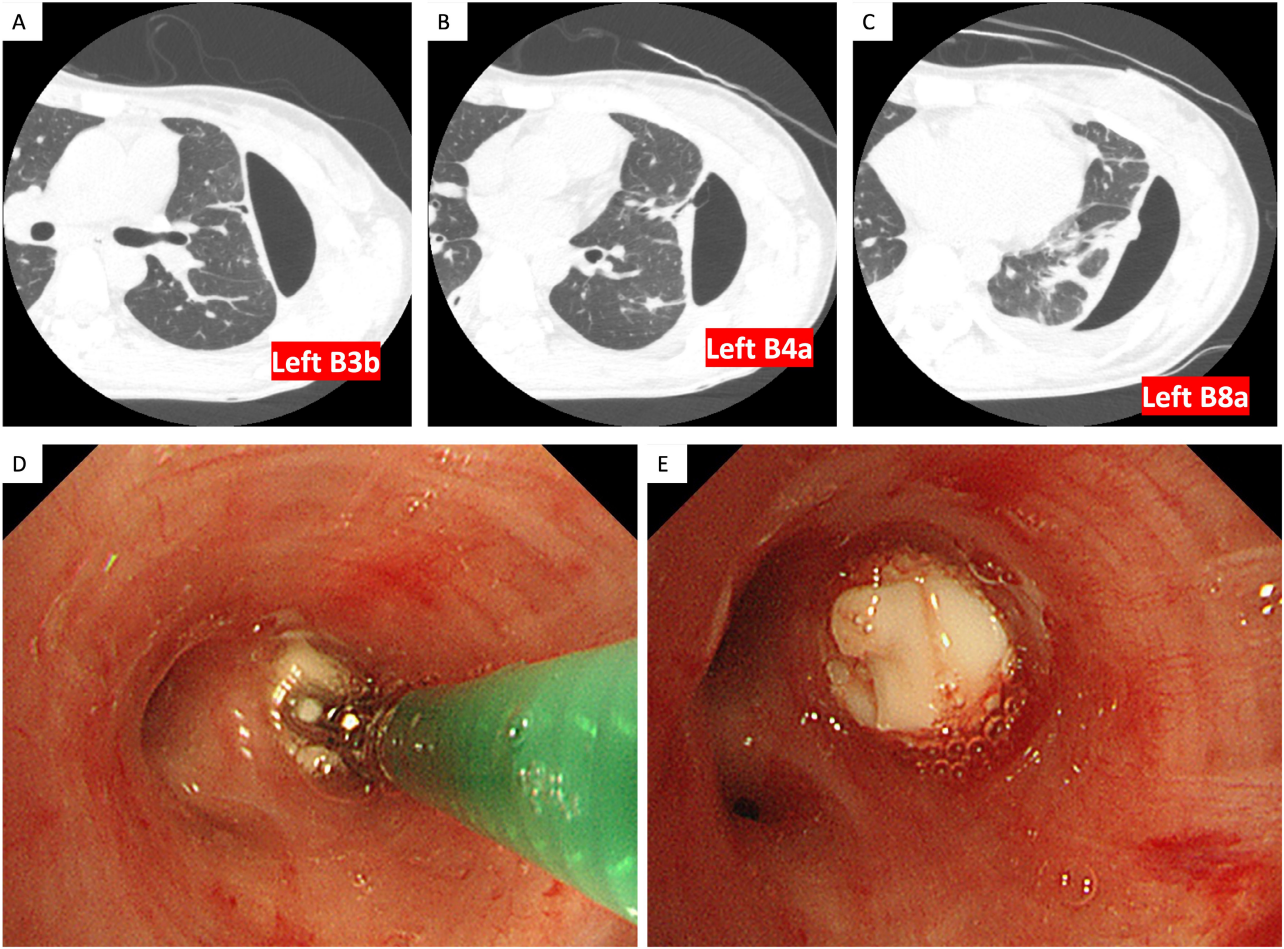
Identification of responsible bronchi and bronchoscopic EWS placement. (A–C) High‐resolution CT (HRCT) on hospital day 36 clearly identified the bronchi responsible for the pneumothorax: left B3b, B4a, and B8a. (D, E) Bronchoscopic findings during EWS placement. EWS were placed in the left B3b, B4a, B8a, and right B10c bronchi.
